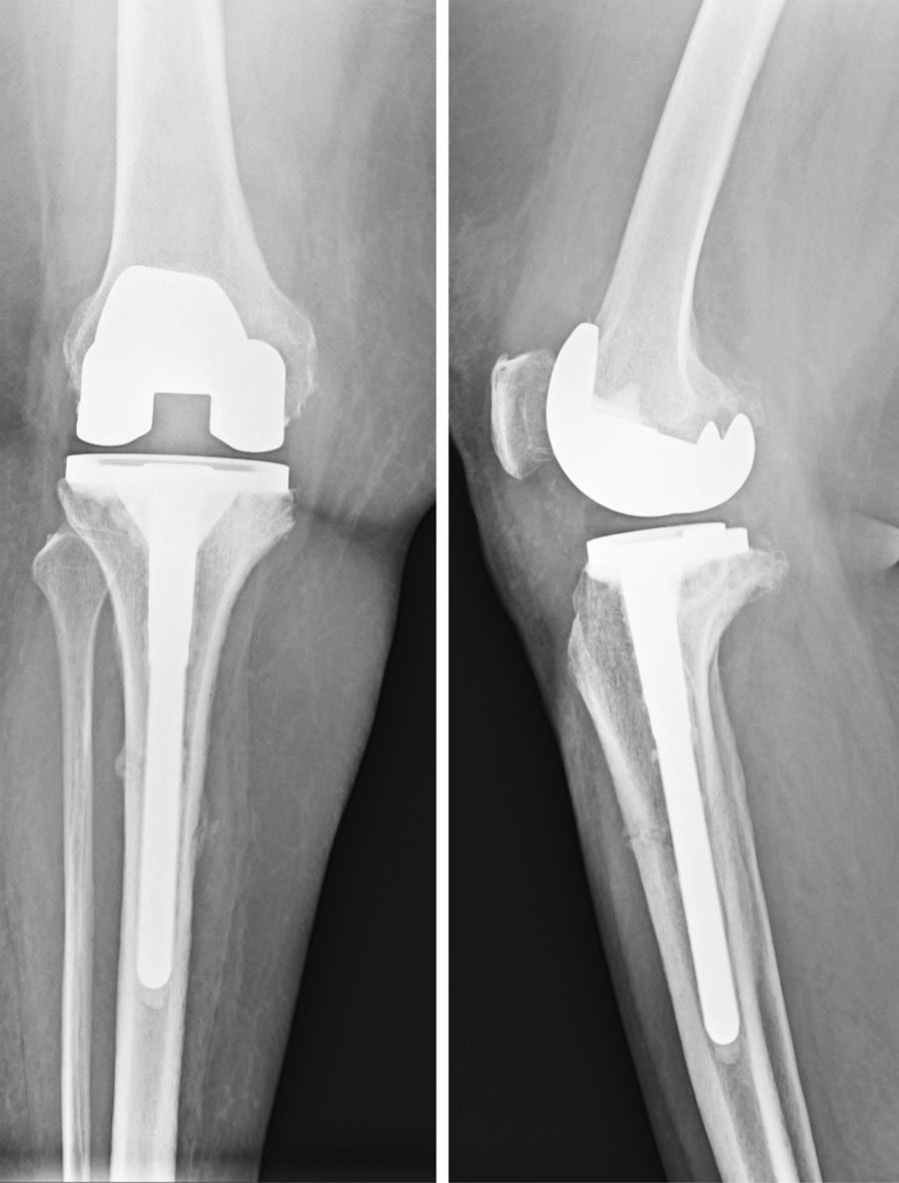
Postoperative 12 month follow-up radiographs of case 2 operated with total knee replacement using long stem extender on tibial side. Notable fracture healing is evident by periosteal new bone formation
